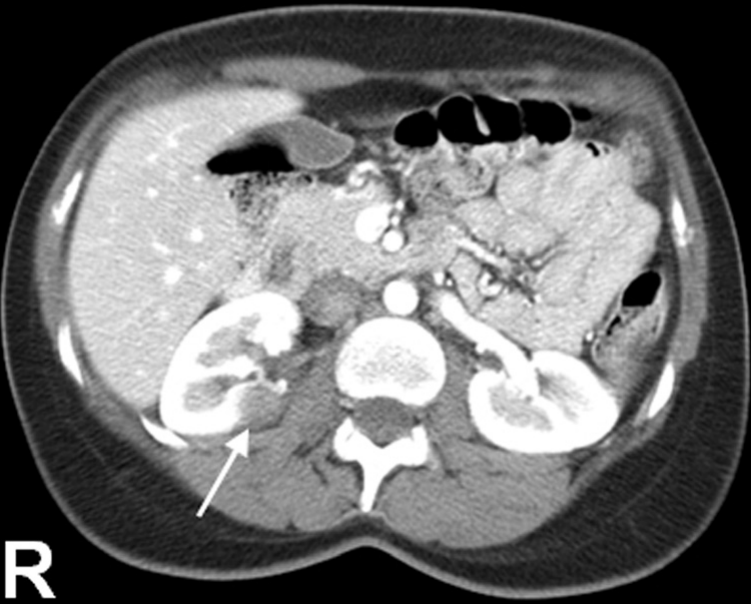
Contrast computed tomography scan of abdomen demonstrating right renal mass with calcifications (arrow). R indicates right side.
